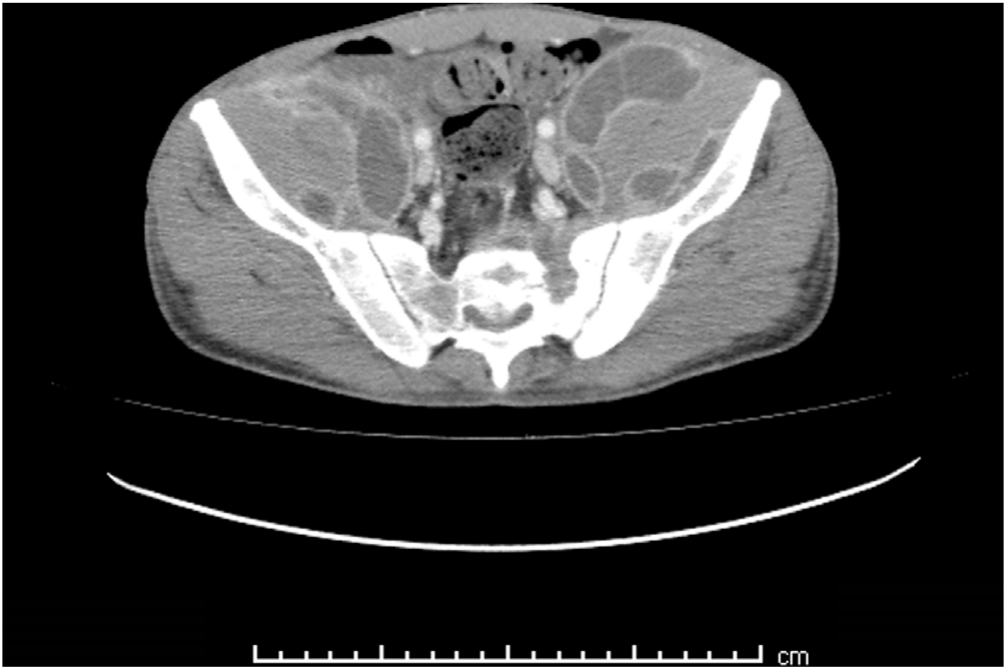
CT-scan axial view: large abscesses in both psoas muscles and multiple bone lytic lesions of the pelvis.
